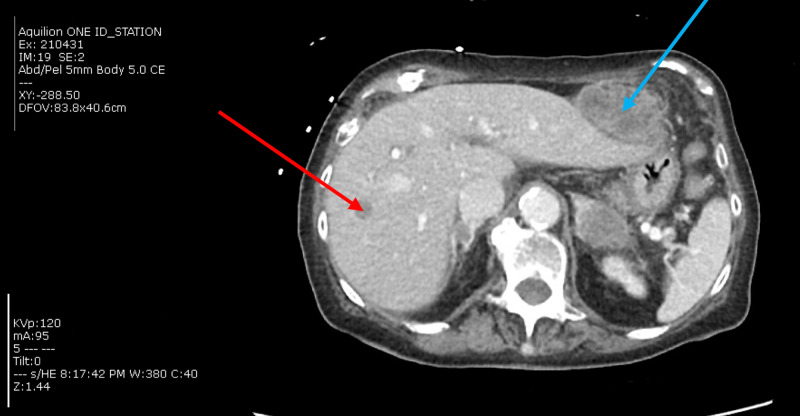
CT abdomen and pelvis shows a large mass (blue arrow) either abutting or arising from in segment 2 of the liver measuring 5.1 cm by 4.0 cm by 6.5 cm with central low attenuation consistent with metastasis. There is another low-attenuation lesion in segment 7 measuring 2.6 cm by 1.2 cm by 1.7 cm (red arrow). Although these may represent simple cysts, metastases are considered more likely
